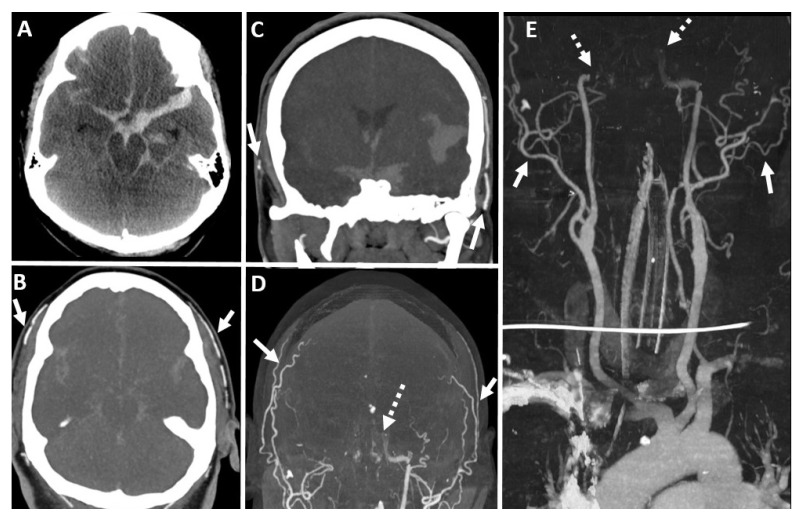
(A) Unenhanced axial CT brain image, (B) axial MIP (maximum intensity projection), (C) coronal MIP, (D) 3D coronal reconstruction of head, and (E) 3D coronal reconstruction of CT neck angiography after an injection of 80 mL contrast iopamidol injection 76% (Isovue 370, Bracco) in a patient with BD/DNC. There is diffuse subarachnoid hemorrhage with cerebral edema (A). CT head and neck angiography demonstrate the contrast opacification of bilateral external carotid arteries and its branches (solid white arrows in (B–E)). The contrast opacification of the bilateral internal carotid artery up to the petrous segments (dashed white arrows in (D,E)). The absence of the contrast opacification of intracranial carotid and vertebral arteries. There is an artifact from endotracheal tube in the reconstructed image of the neck.
